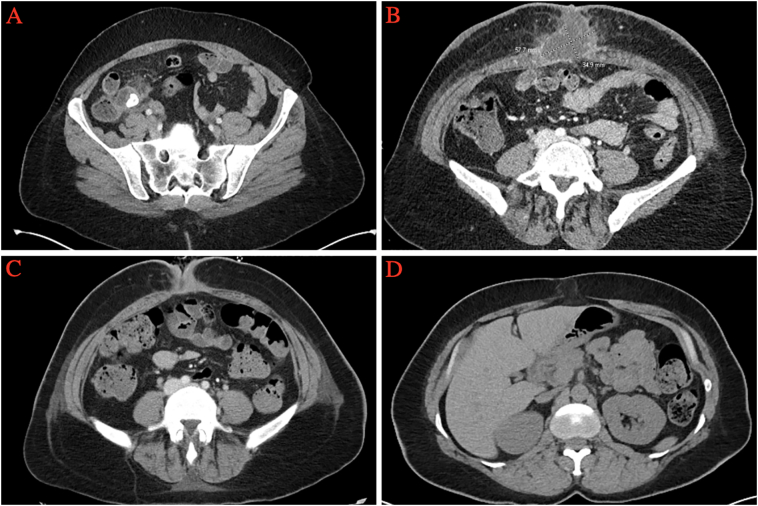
CT abdomen and pelvis with contrast. A: Enlarged appendix surrounded by inflammatory changes and a large luminal appendicolith consistent with uncomplicated AA. B: 5.8 × 3.5 cm fluid collection in the subcutaneous tissue deep to the umbilical port site incision associated with diffuse subcutaneous edema and fat stranding consistent with a laparoscopic port site wound infection. C: Post I&D imaging without evidence of an intra-abdominal fluid collection or abscess. D: A small fat-containing hernia at the laparoscopic trocar site.
